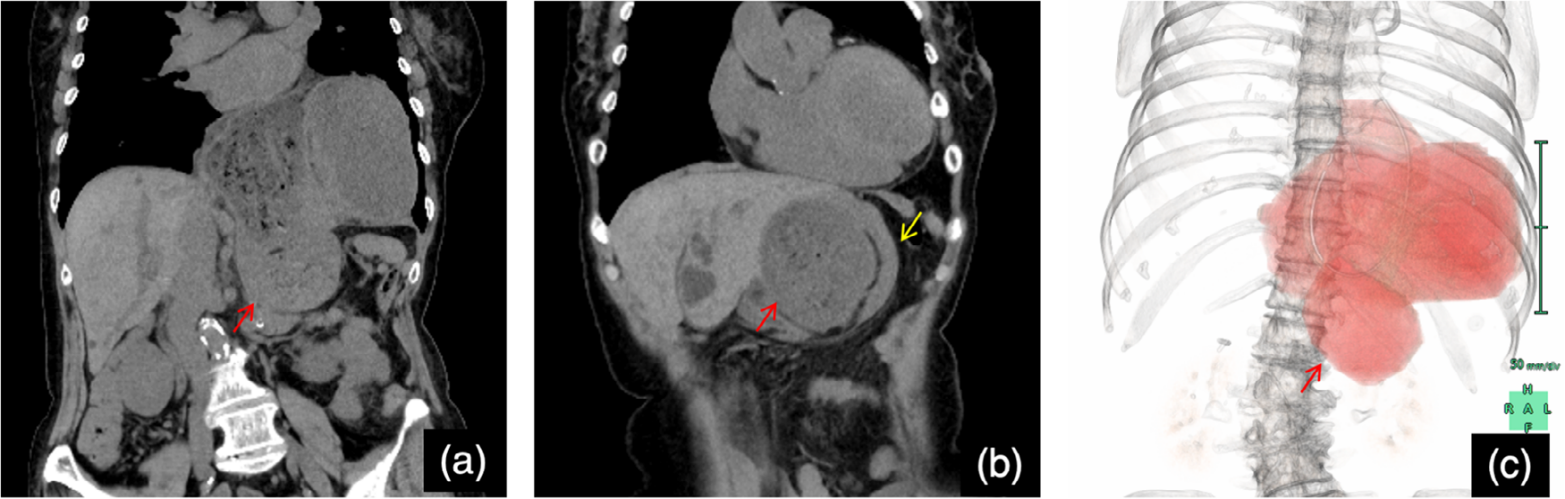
Computed tomography findings on initial admission (a–c) Computed tomography shows hiatal hernia and incarceration of a part of the gastric body into the abdominal cavity. The red arrows show the incarceration of a part of the gastric body into the abdominal cavity, and the yellow arrows show the compressed duodenum.
